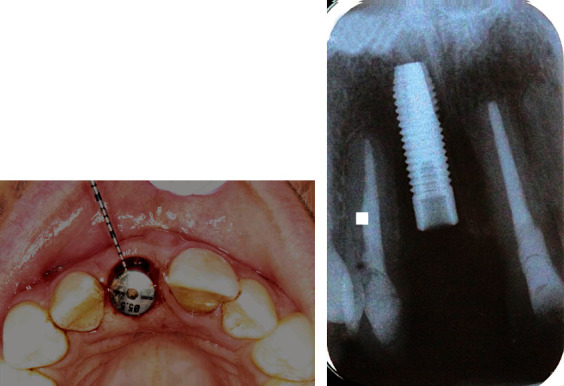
(a) A 5.5 mm diameter healing abutment was connected to an OsseoSpeed 4.0 × 15 mm implant at the position of tooth 11. (b) A periapical radiograph was taken to validate that the implant shoulder was located at least 3 mm apical to the CEJ of the adjacent teeth.
